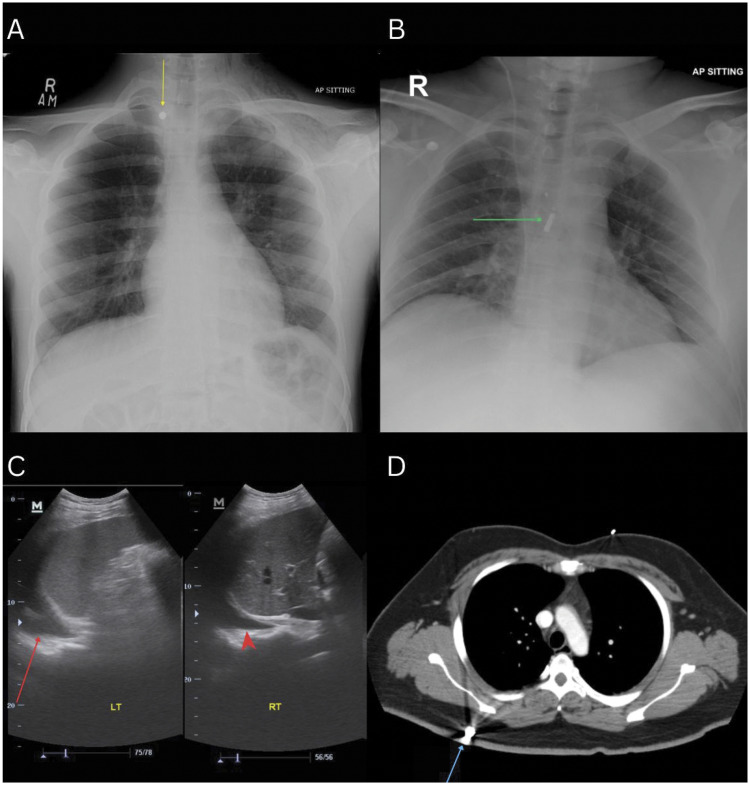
Multimodality imaging of thoracic and associated injuries in bomb blast victims. (a) AP CXR shows a hyperdense metallic foreign body (yellow arrow) overlying the medial aspect of the right clavicle. Subcutaneous emphysema is visible along the left thoracic wall, and a band-like opacity in the left upper lobe is consistent with a pulmonary contusion. (b) AP CXR demonstrating a large metallic foreign body (green arrow) projected over the thoracic spine. (c) Focused Assessment with Sonography in Trauma (FAST) of the left upper quadrant revealing left-sided pleural effusion (red arrow), with the second imaging assessment demonstrating a right-sided pleural effusion (red arrowhead) (d) axial contrast-enhanced CT image of the chest demonstrating distinct metallic foreign bodies (blue arrows) lodged within the subcutaneous soft tissues of the right posterior chest wall. CT: computed tomography; AP: anteroposterior; CXR: chest X-ray.
